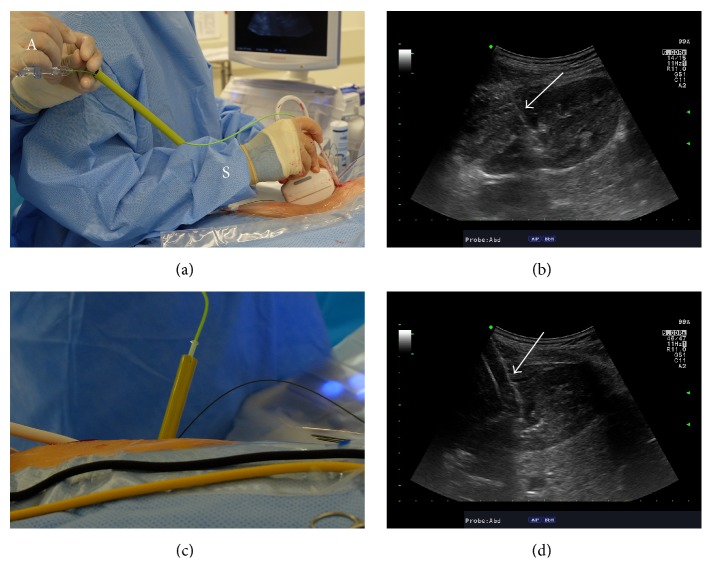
Tract dilation with high-pressure balloon under ultrasound guidance. (a) The deflated balloon dilator is inserted over a working wire and for this step, the operative surgeon (S) controls the ultrasound probe and distal end of the balloon while the assistant (A) controls the wire on the proximal end of the balloon dilator. (b) Ultrasonographic image of the kidney along its longitudinal axis demonstrates that the tip of the deflated balloon dilator (white arrow) is difficult to visualize and differentiate from the wire. (c) The sheath has been inserted over the inflated balloon dilator that is subsequently withdrawn. (d) The inflated balloon (white arrow) can be readily seen with ultrasound imaging.
